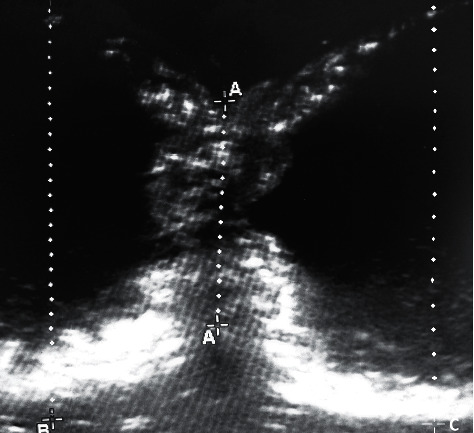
Ultrasound image recorded for luminal diameter reduction. The “*A*” dotted line is at the location of the suture line, the “*B*” dotted line is positioned 2 cm cranial to the anastomosis, and the “*C*” dotted line is positioned 2 cm caudal to the anastomosis.
